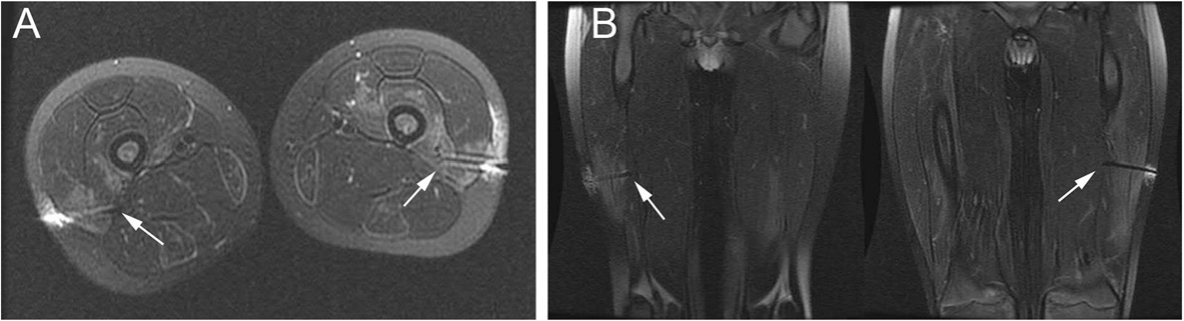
MRI of the needle position within the high T2 signal in the vastus lateralis. (A) Axial and (B) coronal T2 images performed post wire-localization show the localization wires (arrows) are located within regions of high T2 signal in the vastus lateralis muscles bilaterally.
